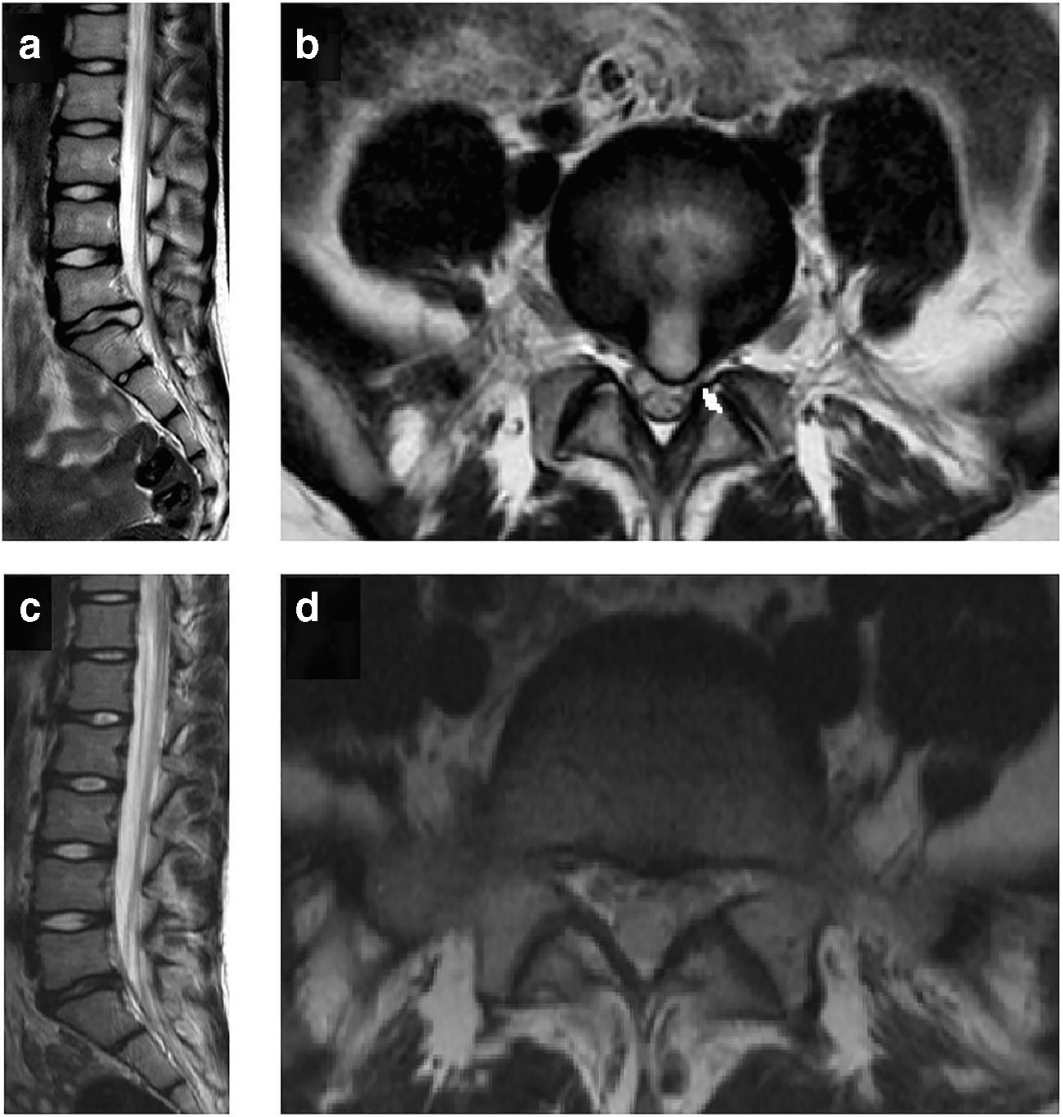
Magnetic resonance imaging showing a median/paramedian lumbar disk herniation at L5-S1 on the left site in sagittal (a) and axial plane (b). c, d the sagittal and axial MRI, respectively, six months after surgery. No residual disk herniation is visible and the nerve root at L5-S1 at the left site is adequately decompressed
